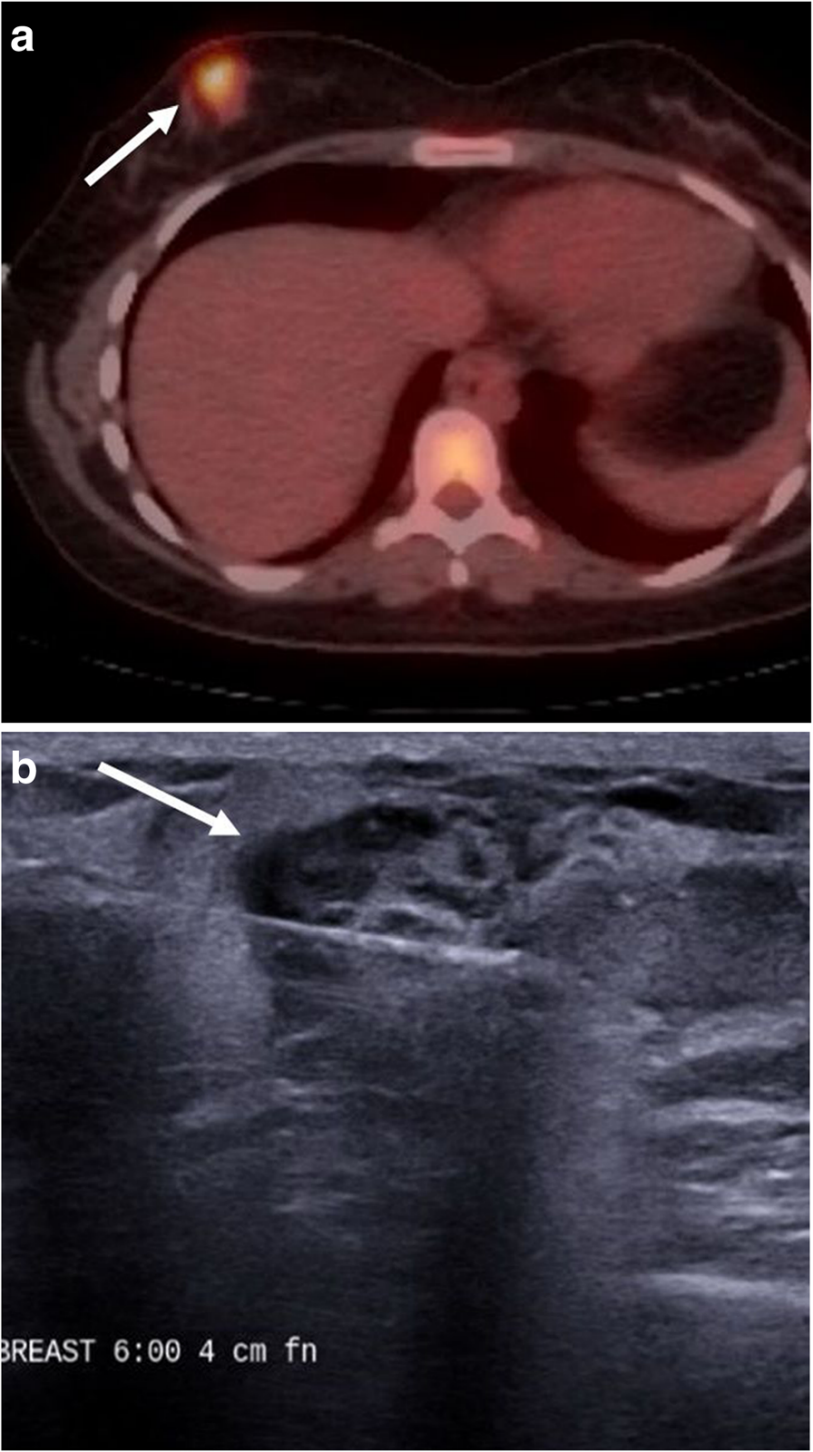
Breast involvement. A 22-year-old female with known Burkitt lymphoma. a Staging fused axial PET-CT demonstrates hypermetabolic activity within the inferior and medial right breast (arrow). b Grayscale ultrasound during percutaneous biopsy demonstrates an irregular, heterogeneous mass with indistinct margins (arrow). Biopsy results revealed Burkitt lymphoma
